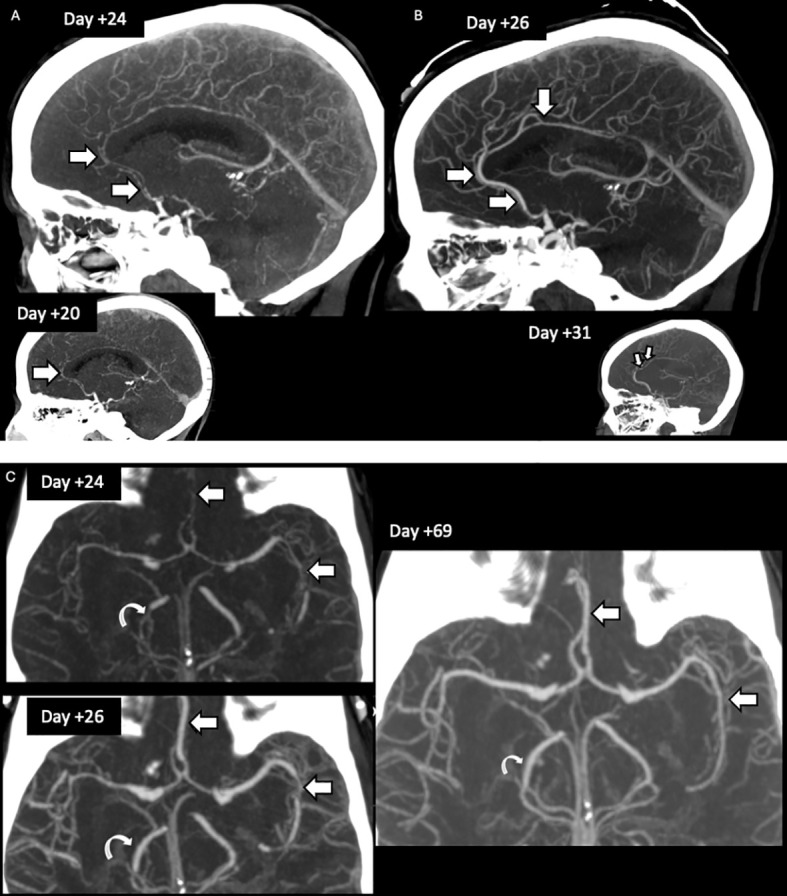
CT angiogram brain/neck. (A) Global vascular irregularity with segmental luminal narrowing’s seen in ACAs (short arrow), both MCAs but more evident in the left MCA (long arrow), and right PCA (curved arrow) as seen on initial presentation CTA Brain and Neck (day +20 to +24). (B) Follow up CTA Brain and Neck shows waxing-waning of luminal irregularities with improved patency of right PCA (curved arrow) and ACAs (short arrow). (C) Post discharge CTA Brain and Neck imaging showing interval resolution of multifocal stenoses in ACAs (short arrow), MCAs (long arrow), and PCAs (curved arrow). ACA, anterior cerebral artery; PCA, posterior cerebral artery; MCA, middle cerebral artery.
